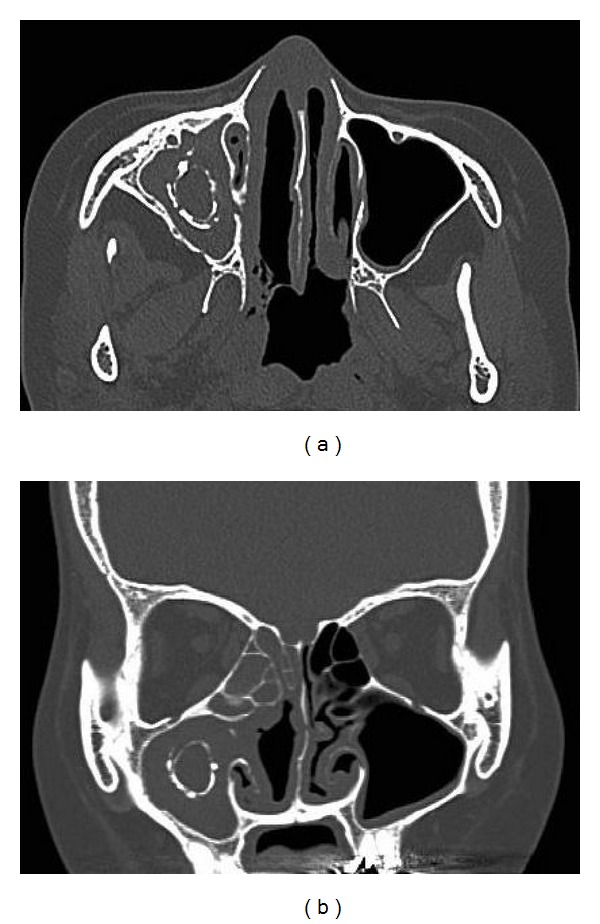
Horizontal (a) and axial (b) CT image. Ring-like calcification is seen in the right maxillary sinus.
